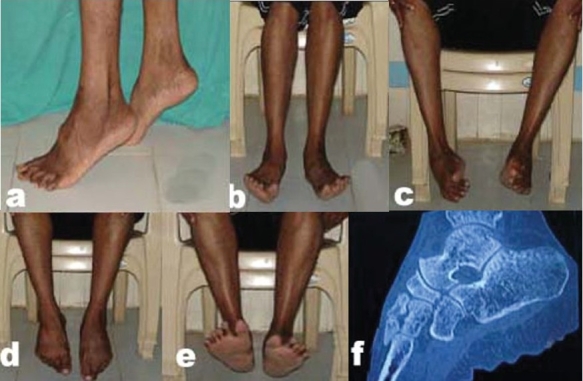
Thirty-two-month follow-up result showing excellent functional (a-e) and radiological outcome. CT scannogram (f), and sagittal CT reconstruction (g) showing congruent subtalar, talanavicular, and calcaneocuboid joints.
